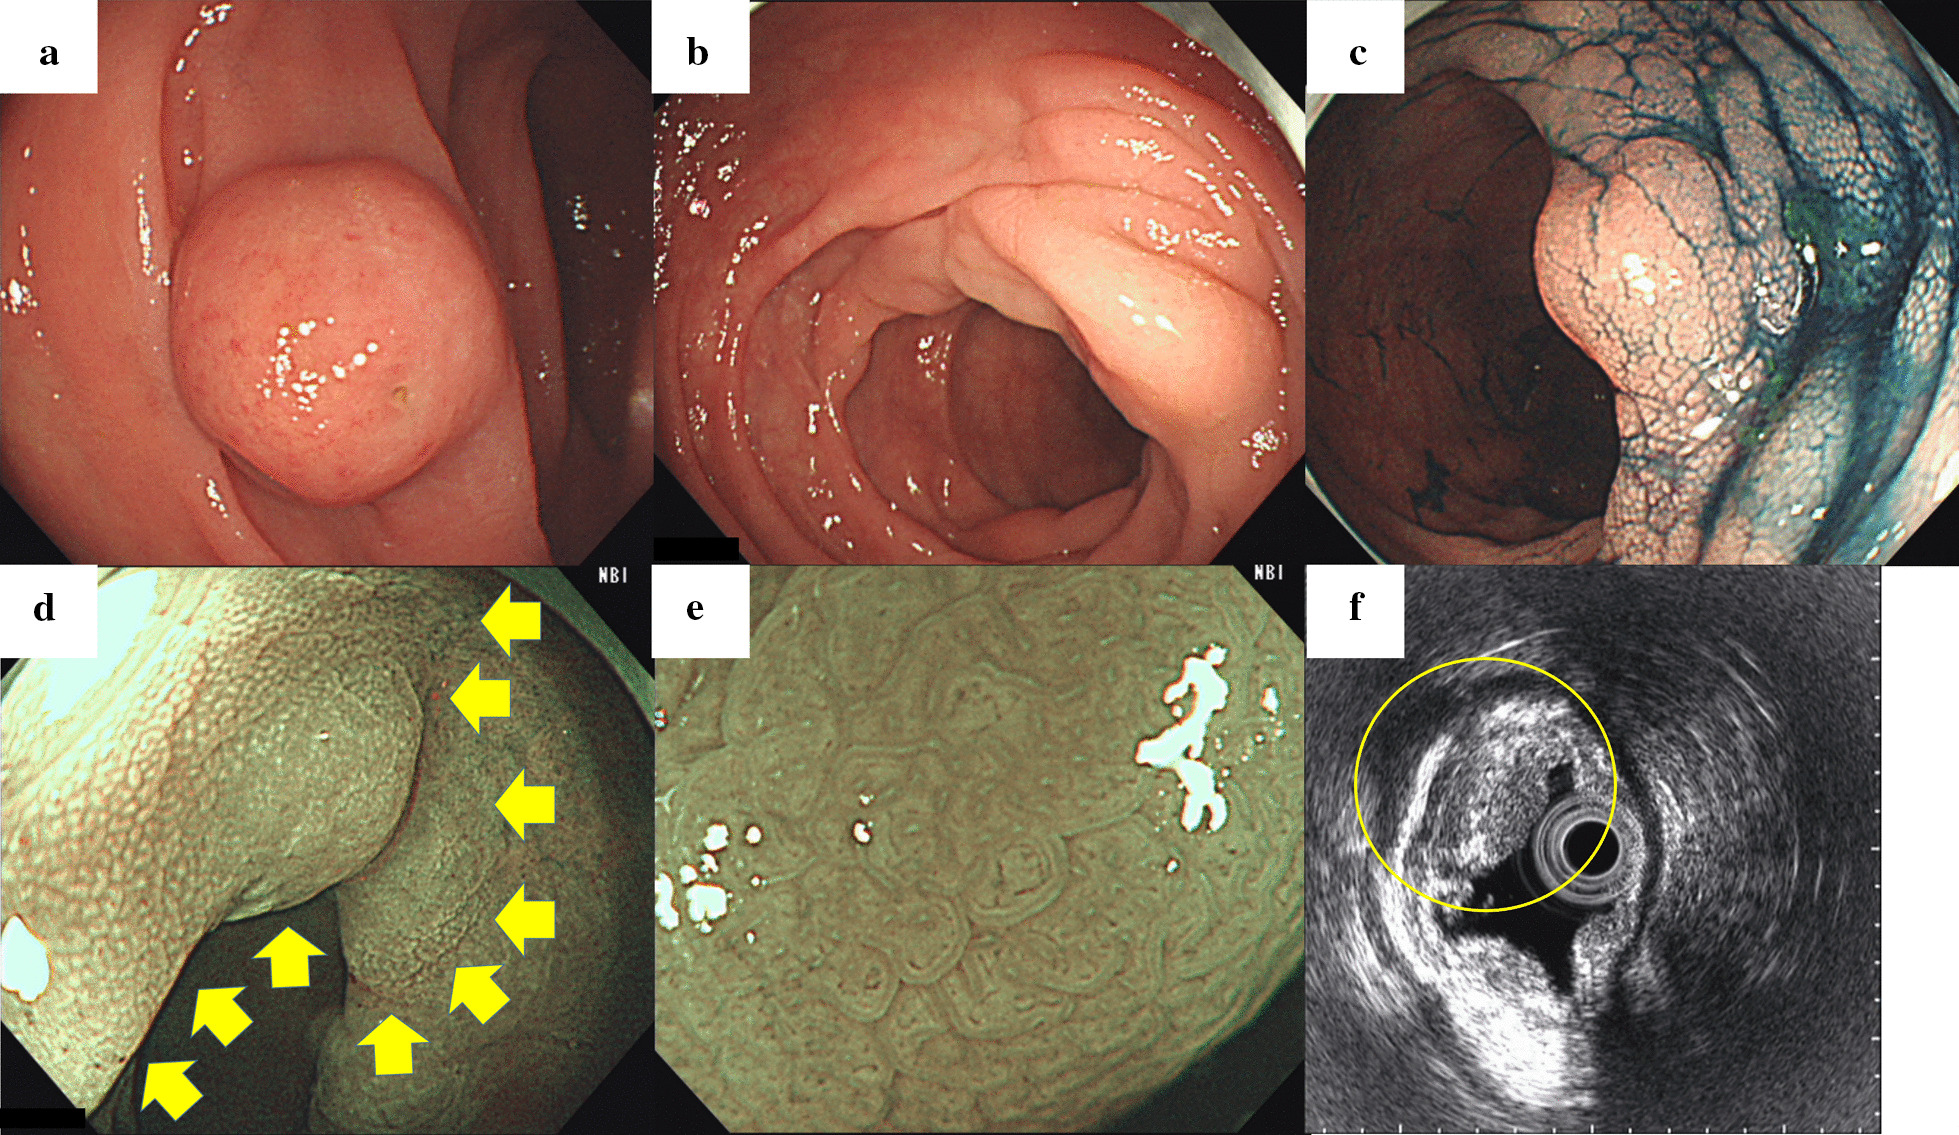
a A subepithelial lesion was observed on initial colonoscopy but subsequently disappeared. b Mucosal Schwann cell hamartomas presenting as numerous edematous elevations and submucosal tumor-like projections were observed throughout the sigmoid colon. c Indigo carmine clearly delineates a submucosal tumor-like projection. d Narrow-band imaging (NBI) of a Schwann cell hamartoma with fine white granular opacities on its surface (yellow arrows). e Magnifying narrow-band imaging revealed elongated crypt openings, increased width of intervening parts, and no visible microvessels. f Endoscopic ultrasound revealed mild, uniformly hypoechoic thickening limited to the first layer (yellow circle)
